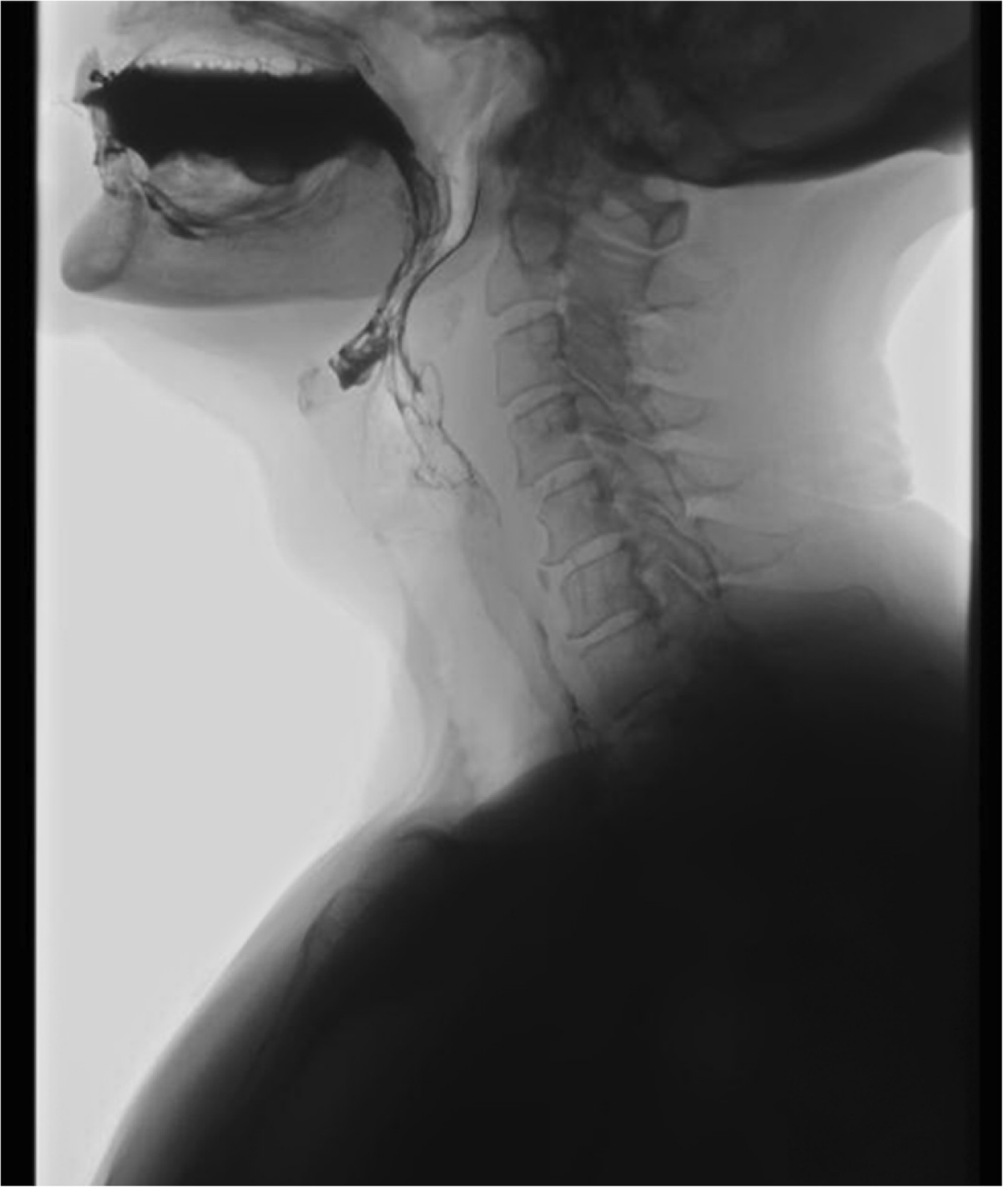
Swallow X-ray with oral contrast. No marked obstruction was visible during the swallow act.
